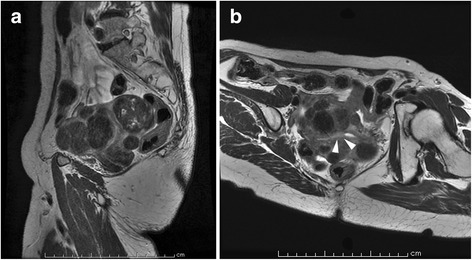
Preoperative magnetic resonance imaging findings of the pelvis show a 2-cm tumor in the uterine cavity (*arrowheads*) and multiple myoma nodules in the uterine corpus
